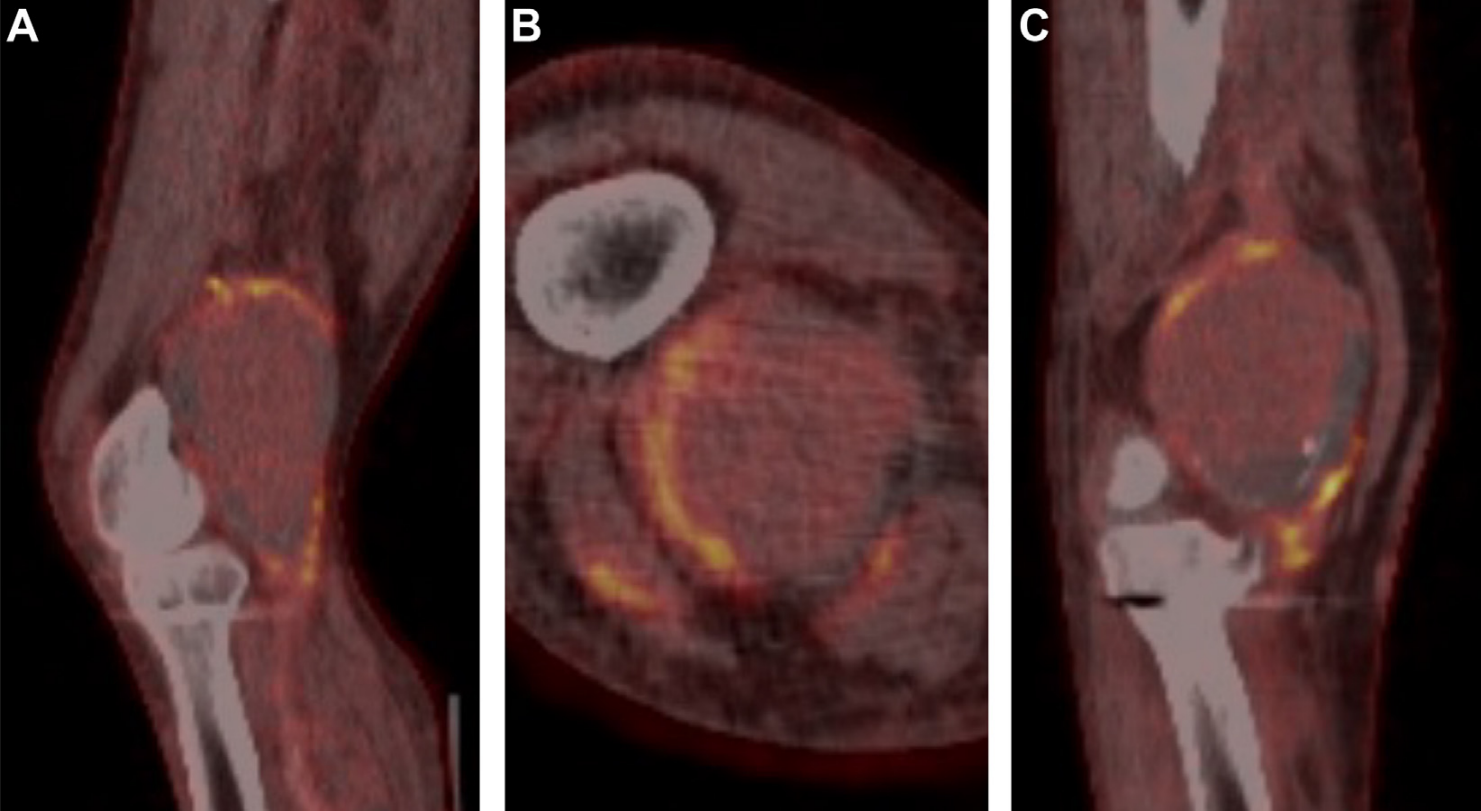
Fluorine-18 fluorodeoxyglucose positron emission tomography/computed tomography images showing hypermetabolic activity on the arterial wall of the large infective popliteal artery aneurysm (PAA) on coronal (A), axial (B), and sagittal (C) projections.
